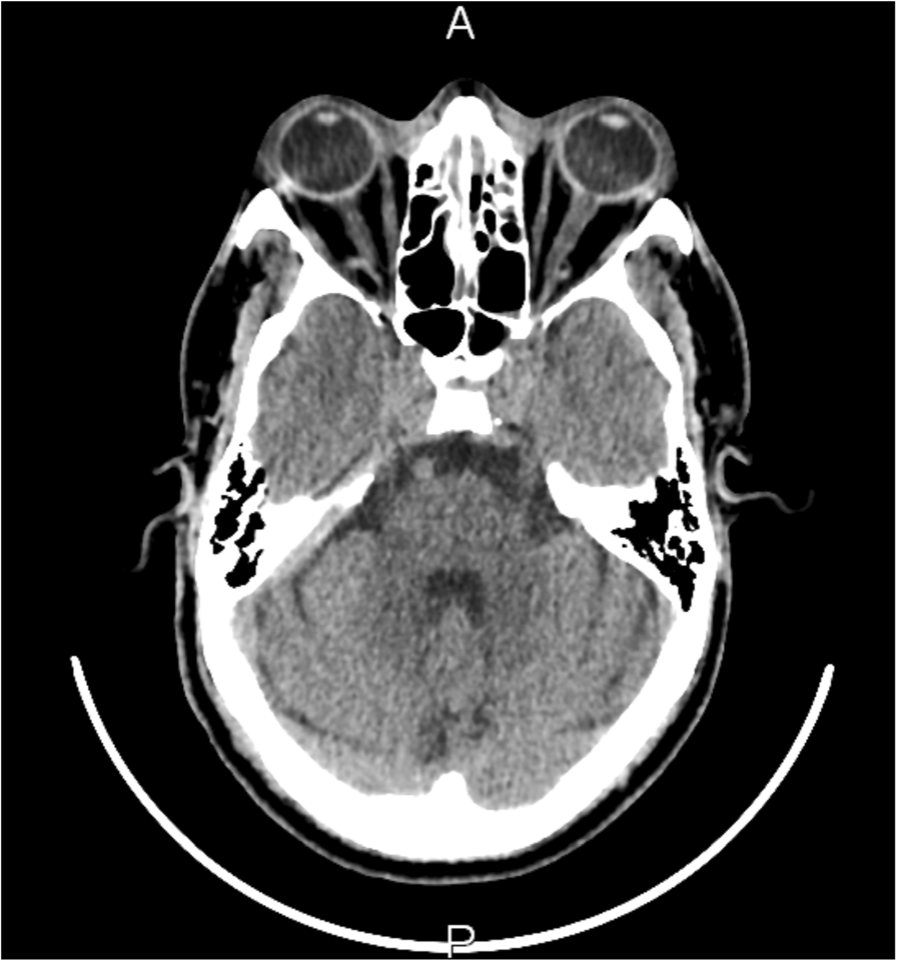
Initial non-contrast CT scan of the orbits, which was reported as unremarkable
